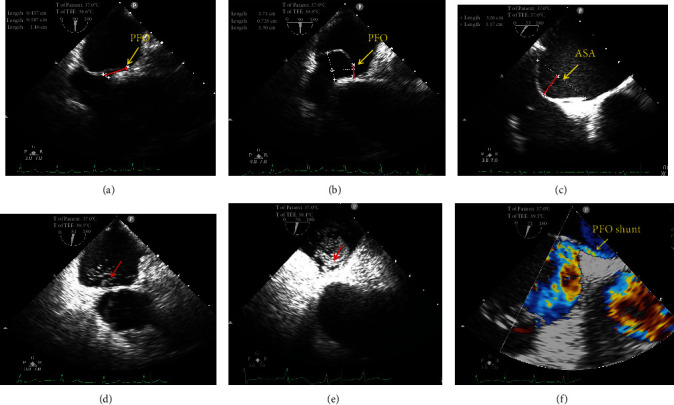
TEE detection of a PFO. (a) The left PFO height and PFO tunnel length were measured at rest on TEE (red lines). (b) The left PFO height after VM on TEE (red line). (c) The atrial septal excursion range on TEE (red line). (d) c-TEE showing a medium RSL through the PFO at rest. (e) c-TEE showing a large RLS through the PFO after VM. (f) Doppler color flow image showing a left-to-right shunt of the PFO. ASA: atrial septal aneurysm; c-TEE: contrast transesophageal echocardiography; PFO: patent foramen ovale; RLS: right-to-left shunt; TEE: transesophageal echocardiography; VM: Valsalva maneuver.
